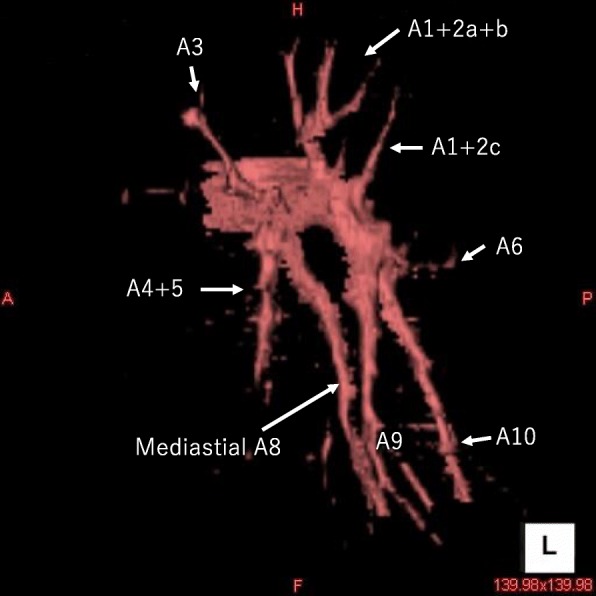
Three-dimensional computed tomographic pulmonary angiography of our case without contrast medium. It was constructed by non-contrast CT retrospectively. It is easy to recognize left mediastinal A8 given off from main pulmonary artery
